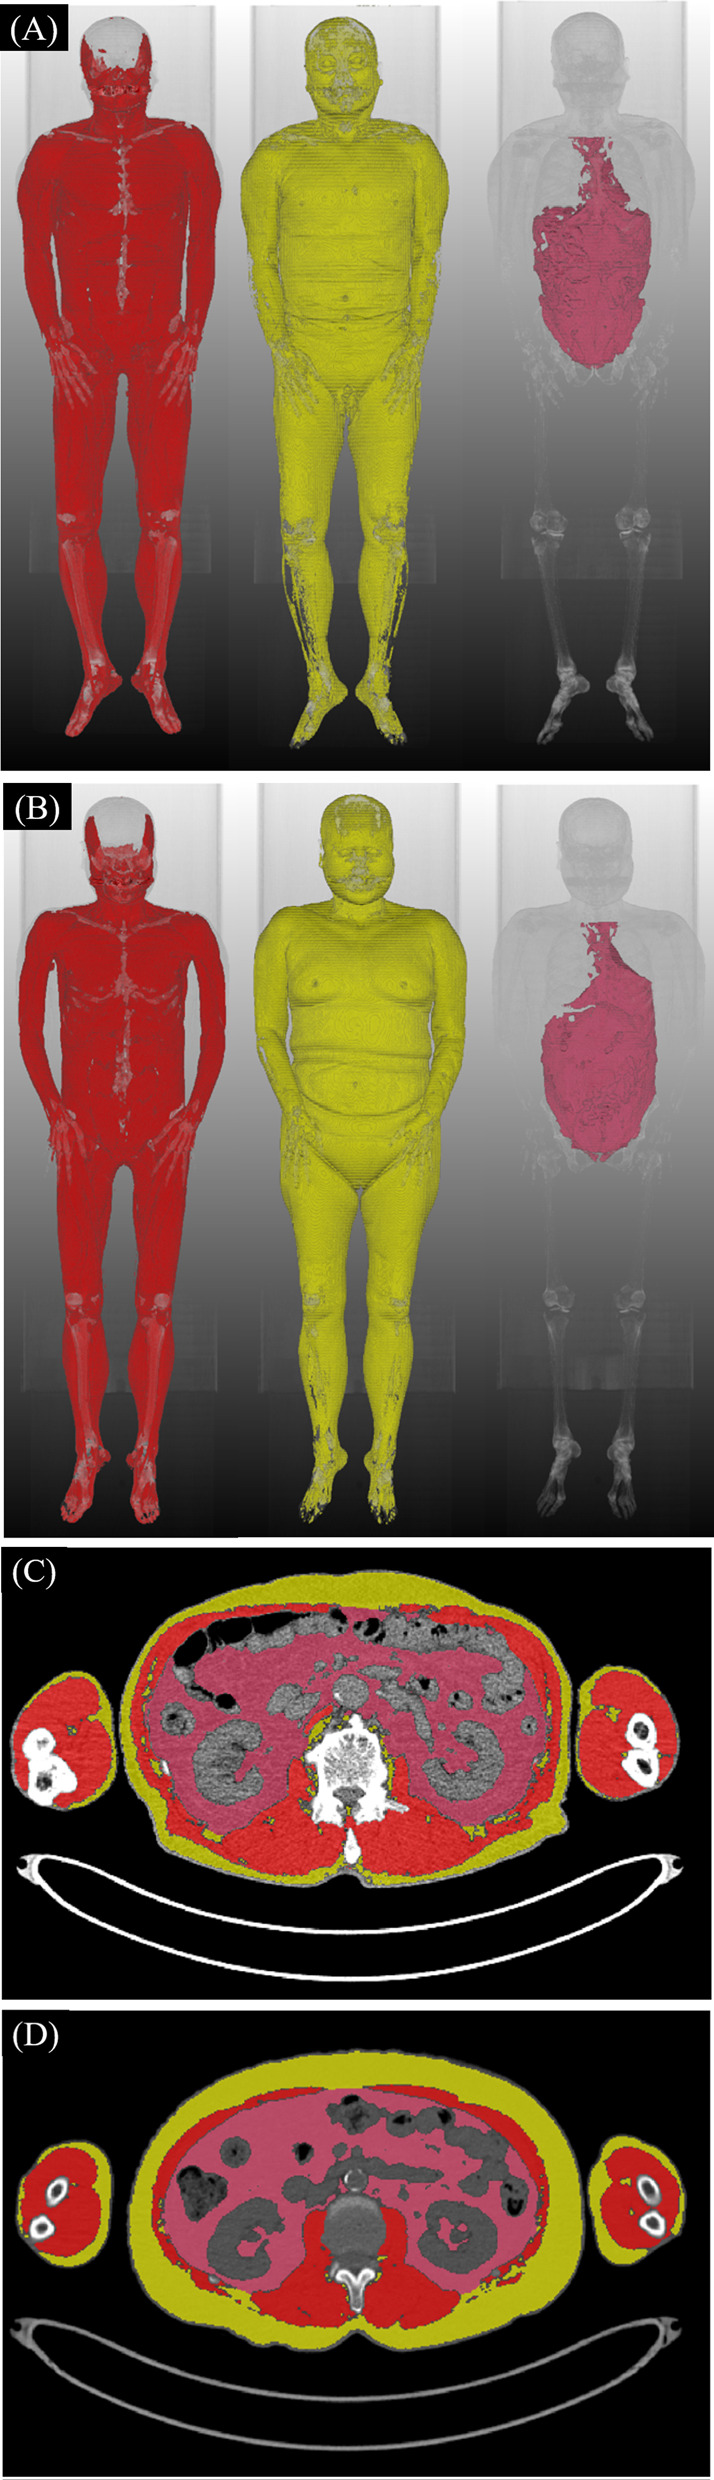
Representative whole-body 3D and cross-sectional CT images of segmented body composition in a 77-year-old male (A, C) and a 68-year-old female (B, D) patients. Whole-body 3D (A, B) and cross-sectional images at the L3 level (C, D) show differences in the distribution of muscle (purple), subcutaneous fat (yellow), and intraabdominal fat (red) by sex. Thoracolumbar cross-sectional areas of muscle, subcutaneous, and visceral fat were assessed from the trunk after excluding those tissues in the arms
